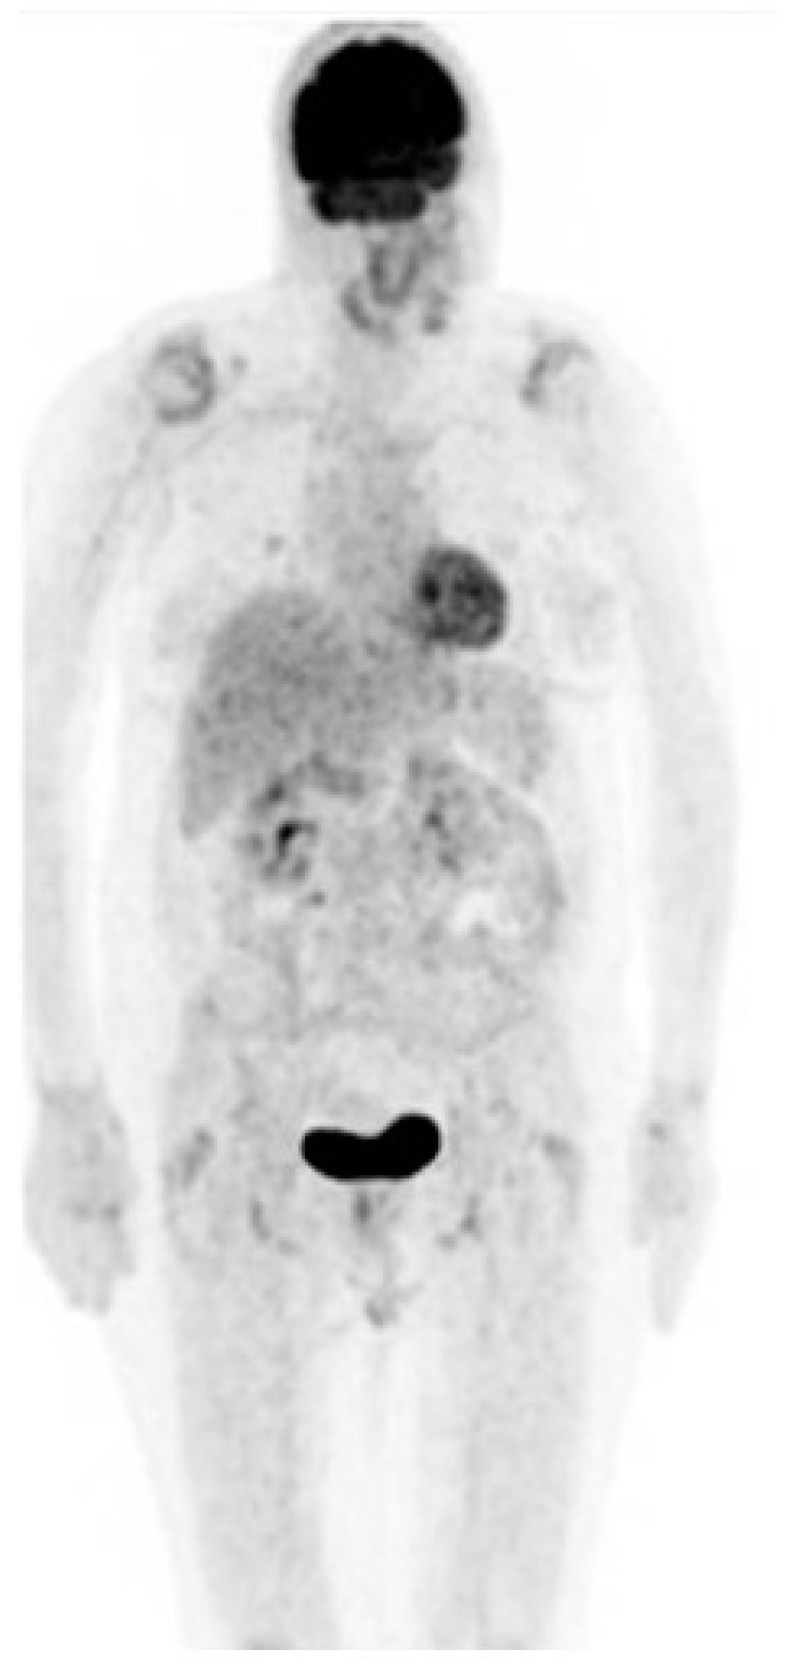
PET–CT scan showing no evidence of disease.
